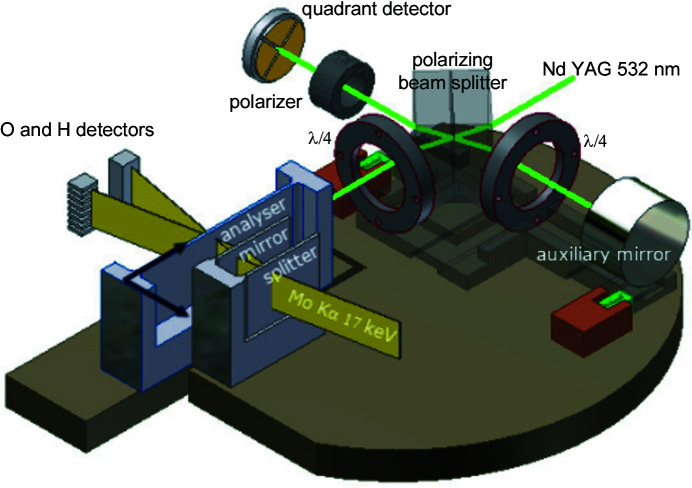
INRIM’s combined X-ray and optical interferometer. The analyser displacement and the pitch and yaw angles are measured by laser interferometry and differential wavefront sensing. The transverse displacements (horizontal and vertical) are measured via capacitive sensors (not shown in the figure). The arrows indicate the positive directions of the axial and out-of-focus displacements.
